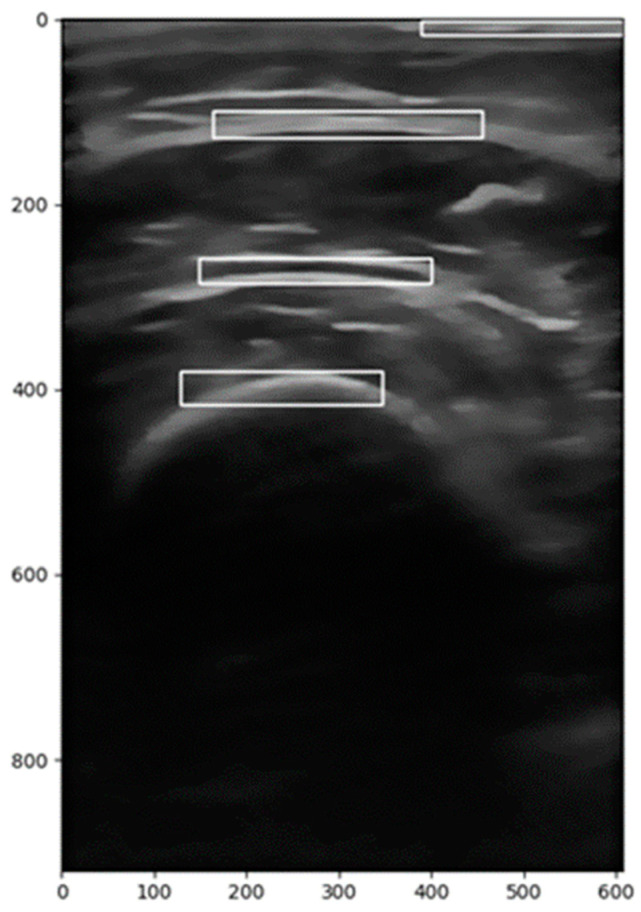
Identified anatomical regions using predefined segmentation templates of ROI (range of interest) in ultrasound images.
